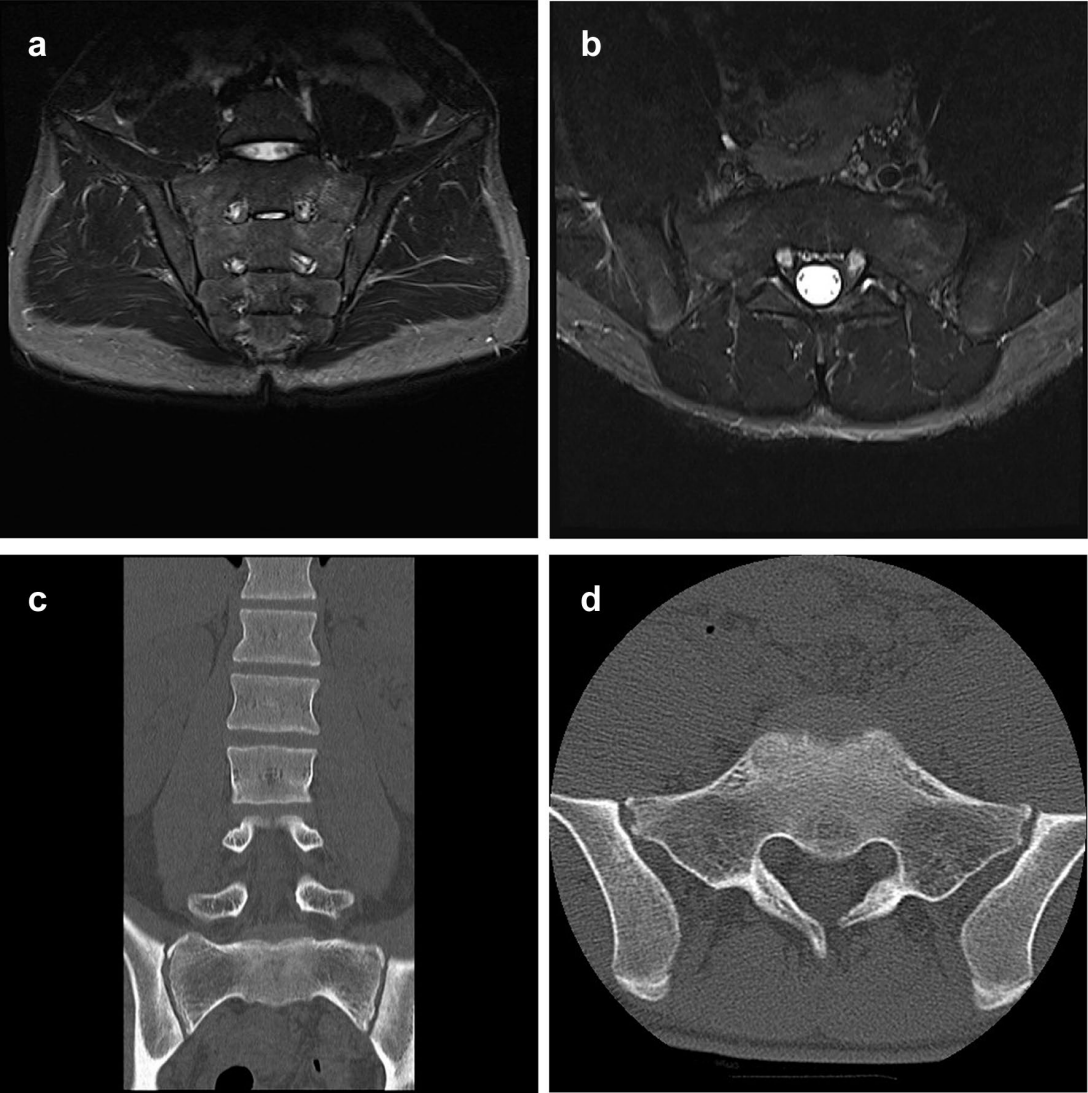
Images after treatment of the representative case. (a) MRI STIR coronal view shows a normal signal on the left sacral alar. (b) MRI STIR axial view shows a normal signal on the left sacral alar. (c) CT coronal view shows no fracture line on the left sacral alar. (d) CT axial view of the sacrum showed no fracture line on the left sacral alar.
